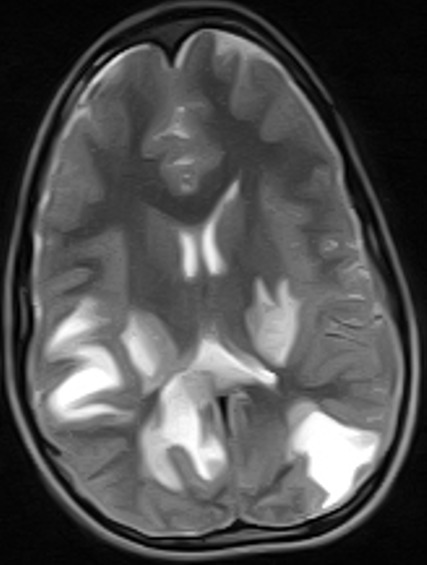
(MRI at presentation) is an axial T2 w sequence showing multifocal, irregular, hyperintense areas in the parietal and occipital regions
